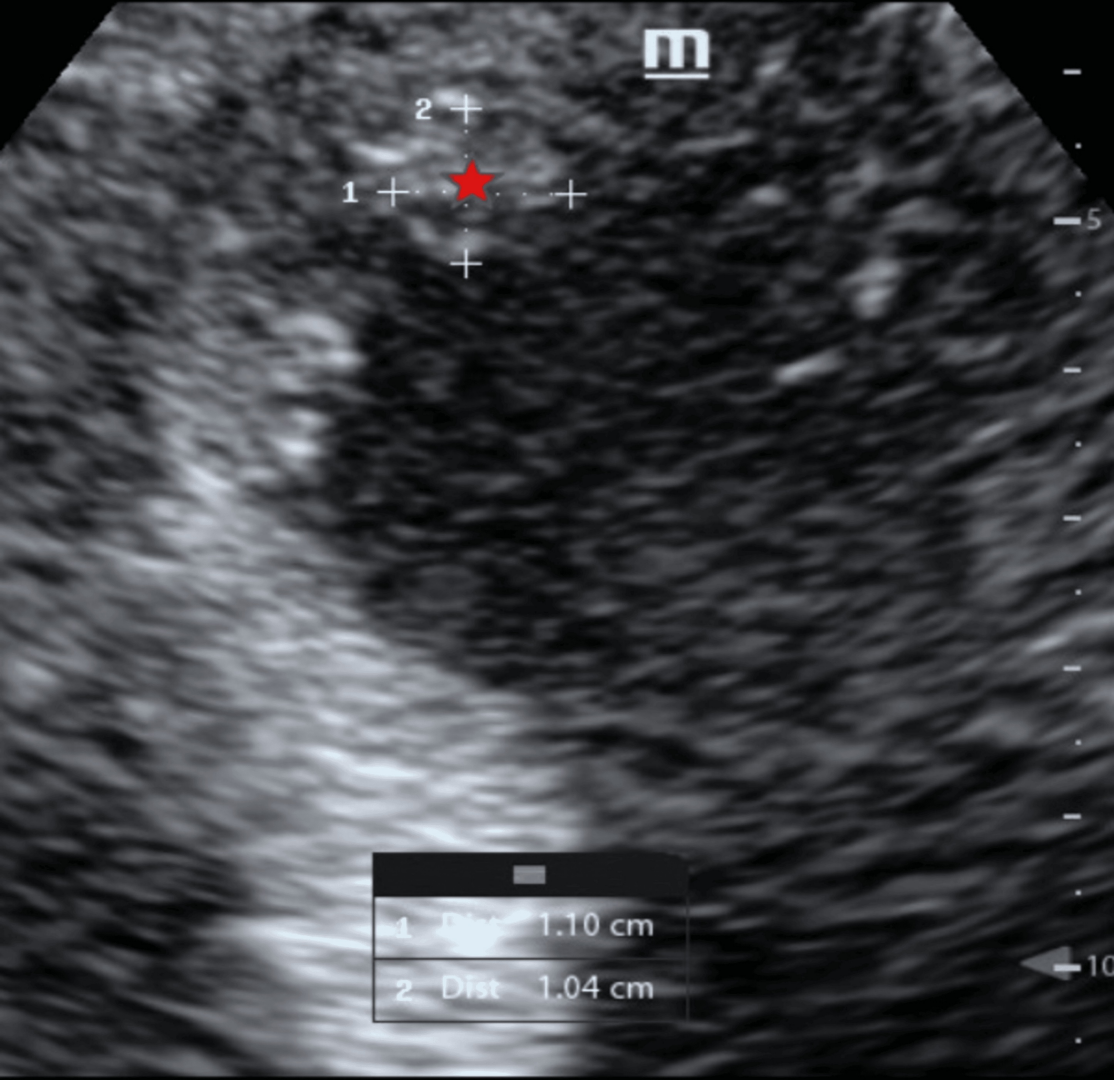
Apical four-chamber view (focused apical imaging) demonstrating a left ventricular intracavitary thrombus Transthoracic echocardiography with focused imaging of the left ventricular apex demonstrating an intracavitary thrombus attached to the endocardial wall, measuring approximately 1.10 × 1.04 cm (red asterisk).
